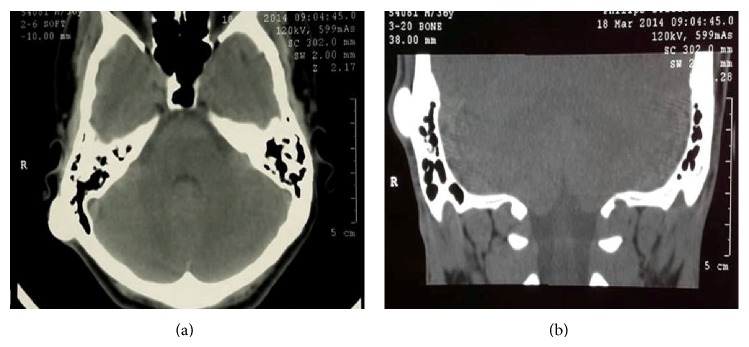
(a) Osteoma in axial cut CT scan. (b) Osteoma in coronal cut CT scan.
